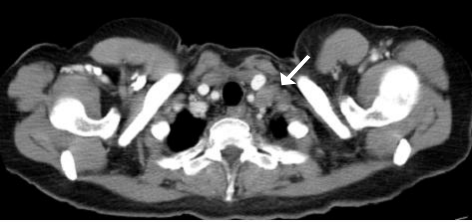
Computed tomography of the thorax, performed postoperatively, shows a 1.6-cm left supraclavicular lymph node deep to the sternocleidomastoid muscle.
